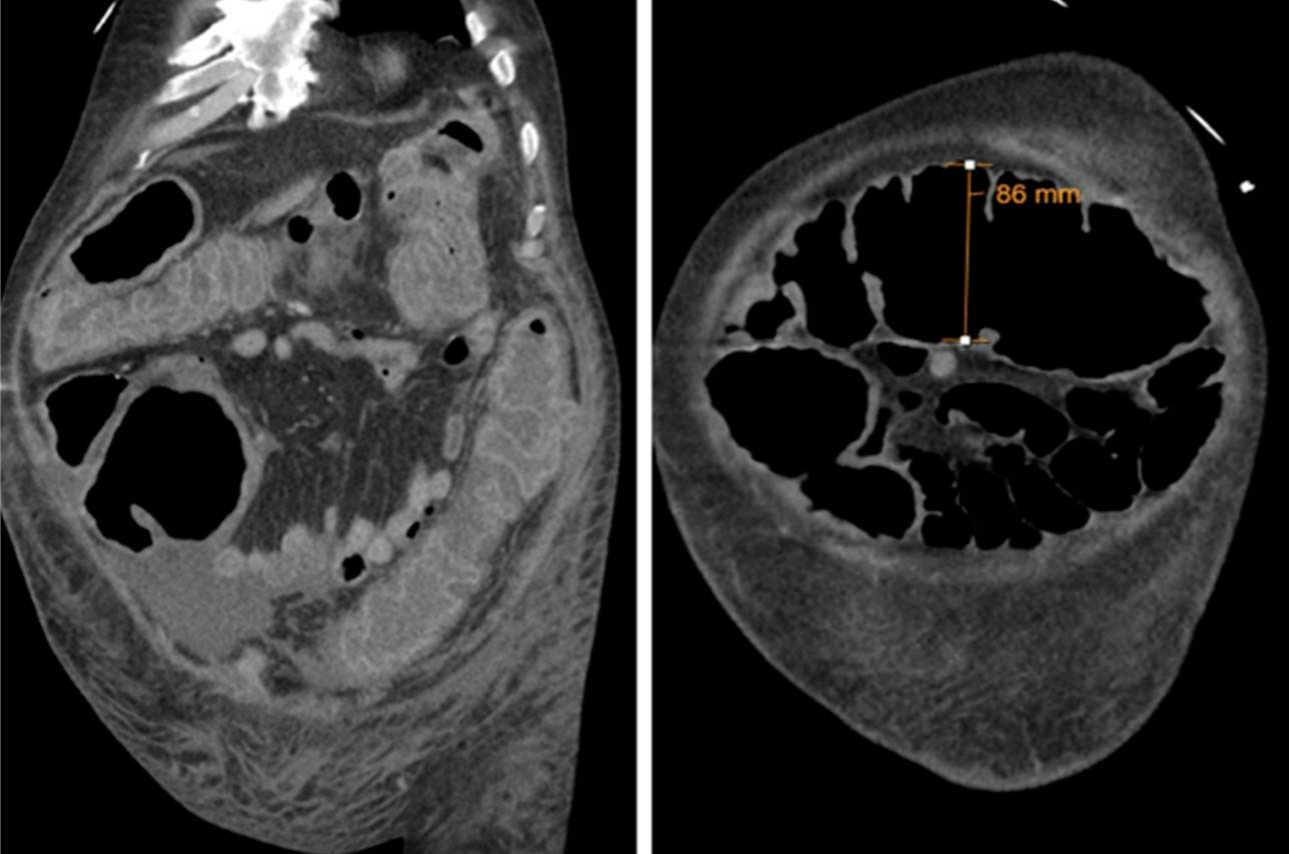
Interval computed tomography of the abdomen/pelvis with colonic dilation and circumferential wall thickening, consistent with toxic megacolon.
